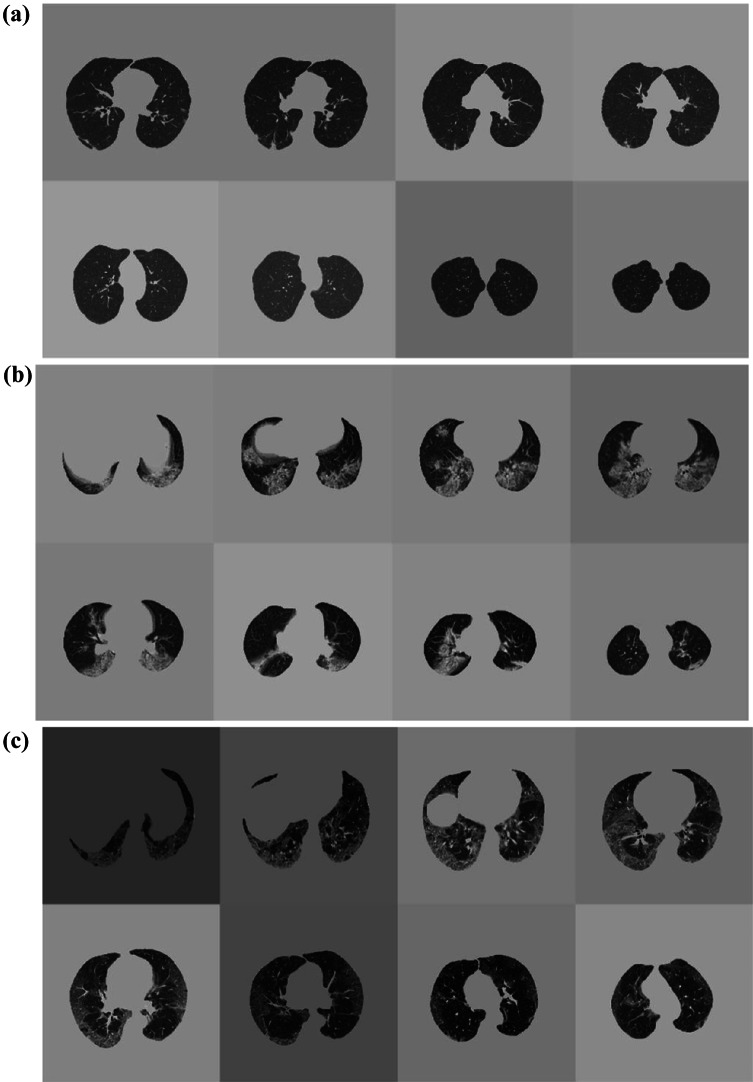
CT examples of patients not-correctly predicted in a survivor, b intubation, and c ICU admission prediction
